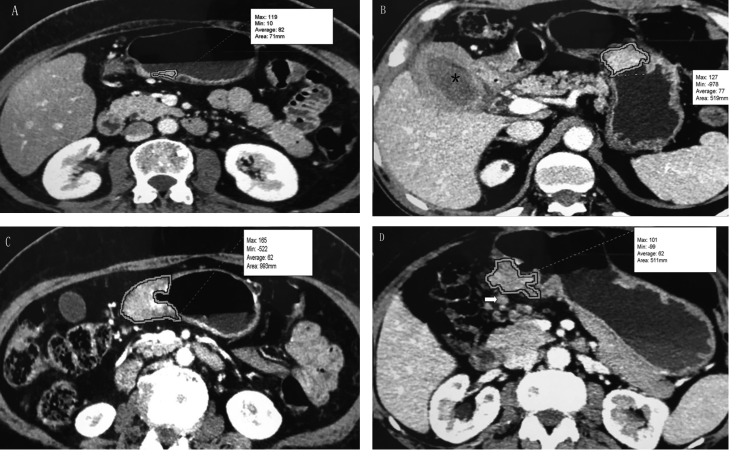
Tumor volume measurement on MDCT (A) T1 stage gastric adenocarcinoma on the gastric angle in a 58-year-old man. Tumor area is manually drawn along margin of tumor, and value of this area (71 mm) is automatically derived by software together with minimal, maximal, and average CT attenuation (in Hounsfeld units). (B) T2 stage gastric adenocarcinoma on the gastric body and xanthogranulomatous cholecystitis (^*^) in a 55-year-old man. The tumor had an area of 519 mm. (C) T3 stage gastric adenocarcinoma on the gastric antrum in a 48-year-old man. The tumor had an area of 993 mm. (D) T4a stage gastric adenocarcinoma on the gastric antrum in a 67-year-old man. The tumor had an area of 511 mm. Lymph node metastasis was found adjacent to the tumor (arrow).
